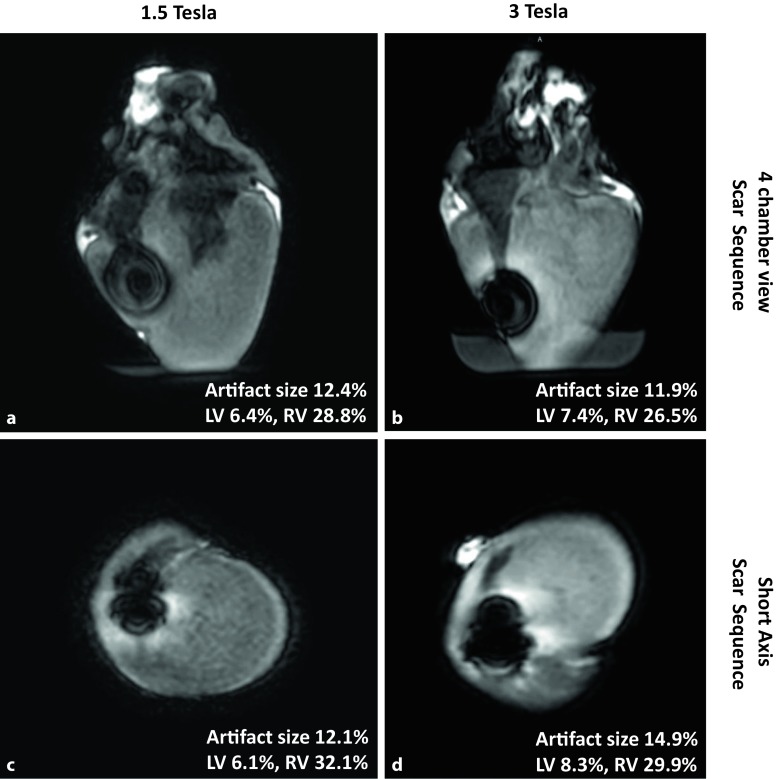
Magnetic resonance imaging scar sequence (a scar sequence at 1.5 Tesla, c short axis view at 1.5 Tesla) most prone to artifacts at 3.0 T, leaving the right ventricle and the septum affected by a bright, hyperintense perifocal rim (b scar sequence at 3 Tesla, d short axis view at 3 Tesla)
